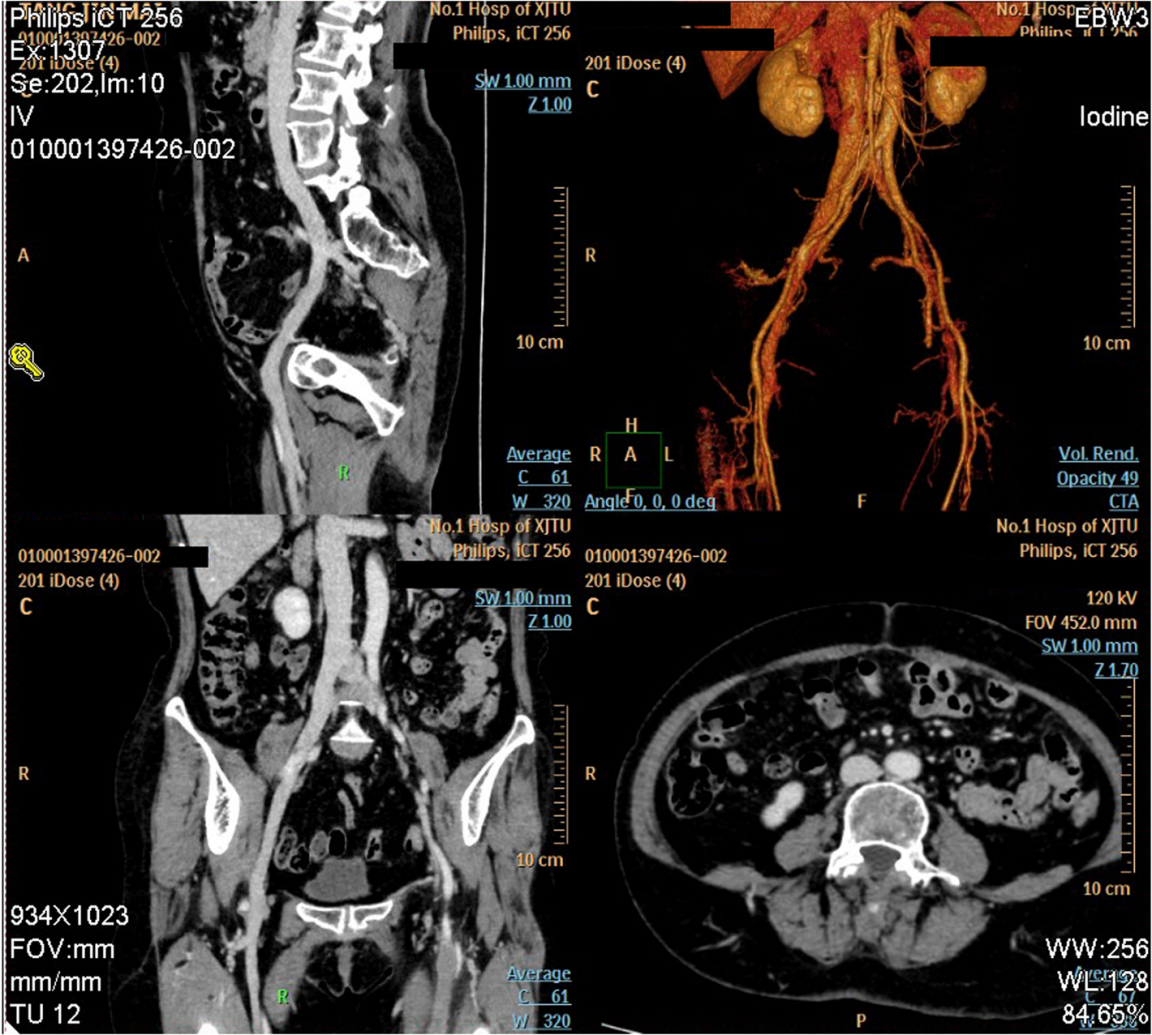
CT arterial angiography before operation
